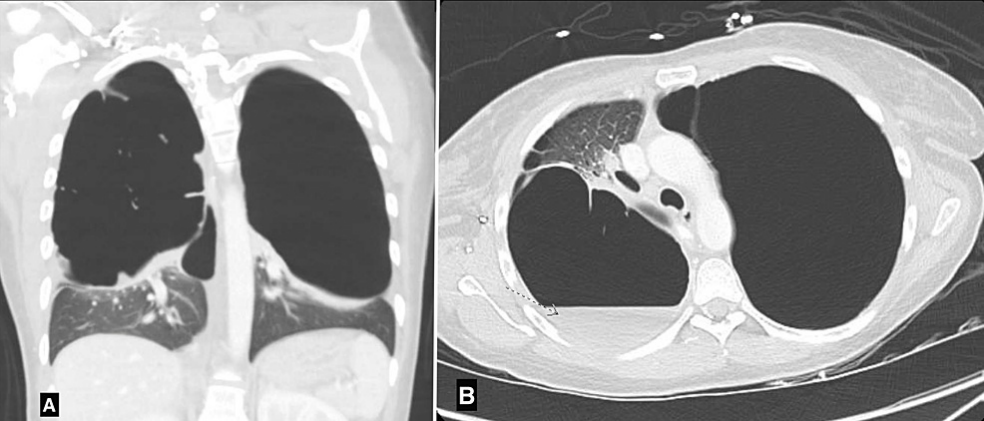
Chest CT scan coronal view (A) and transverse view (B) showing severe bilateral apical bullous emphysema without pneumothorax and an air-fluid level in the right apical bullae (arrow) suggestive of superimposed pneumonia CT: computed tomography
